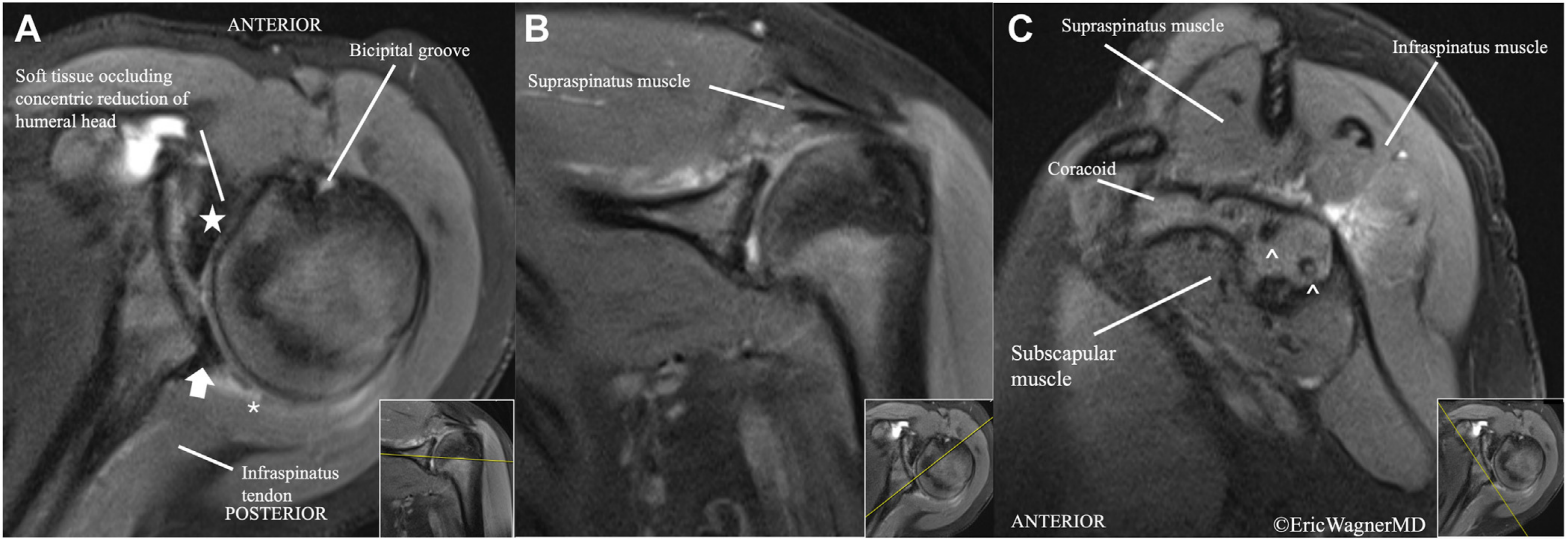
Proton density fat-saturated magnetic resonance imaging of left shoulder in axial (A), coronal (B), and sagittal (C) planes. (A) Axial view demonstrates severe glenoid dysplasia and retroversion with posterior capsulolabral complex detachment and soft tissue interposition in the anterior joint (*star*). Chondral defect at humeral head (∗). (B) Coronal view demonstrates articular defects in location of previous anchor tracks. (C) Sagittal view demonstrates postsurgical anchor tracks in the glenoid from previous capsulolabral fixation (ˆ), and a deficient glenoid.
